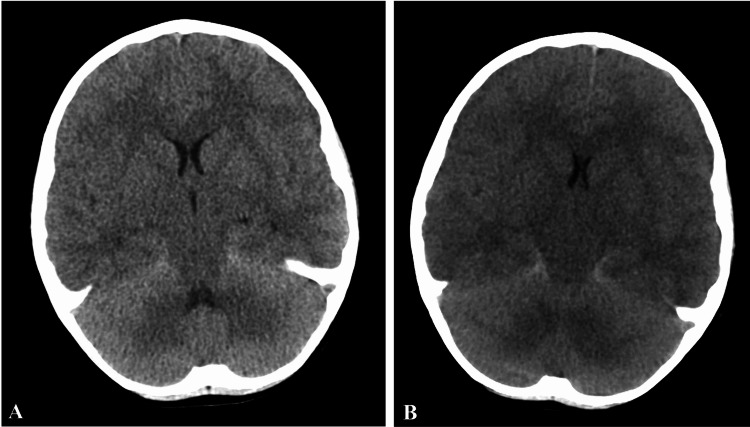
(A) Initial CT scan of the head. (B) Repeat CT scan of the head (A) Initial CT scan of the head demonstrating no acute intracranial hemorrhage, midline shift, or mass effect. (B) Repeat CT scan showing evidence of increased intracranial pressure, without evidence of acute territorial infarction or acute intracranial hemorrhage.
